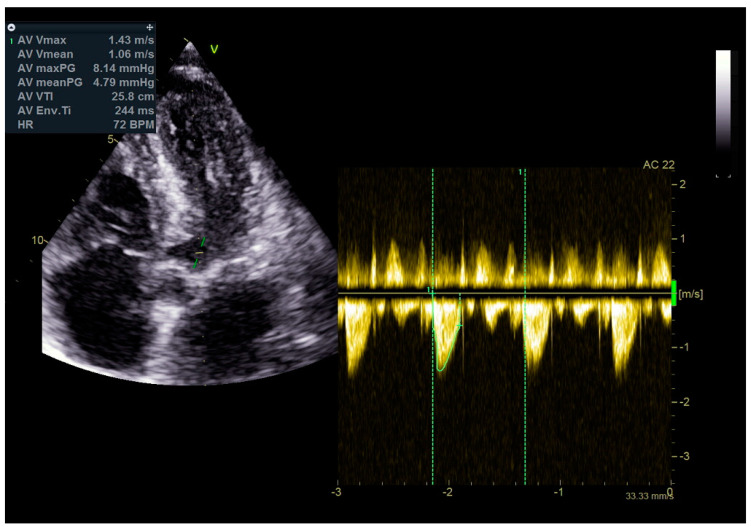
No significant gradients were registered above the LVOT, measured by CW Doppler echocardiography.
